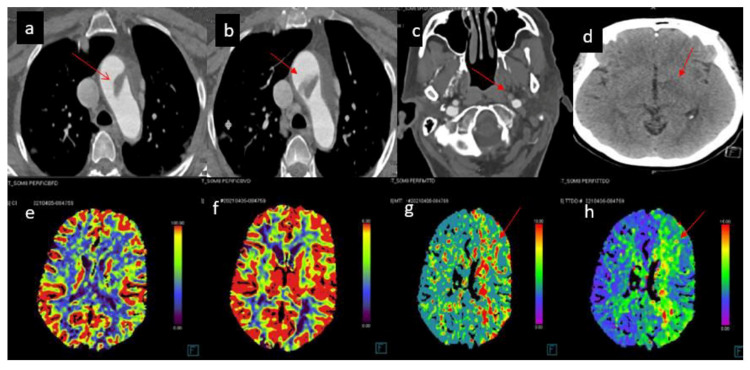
CT head, perfusion and angiography showed a large 2.2-cm floating thrombus within the proximal aortic arch attached to the anterior wall (a, b), and left parietal acute ischemic stroke, left side ICA occlusion and focal severe stenosis at proximal left MCA-M1 segment causing left cerebral hemisphere small infarcted cores, penumbra and other areas of delayed perfusion (c-h). MCA: Middle cerebral artery; ICA: Internal carotid artery.
